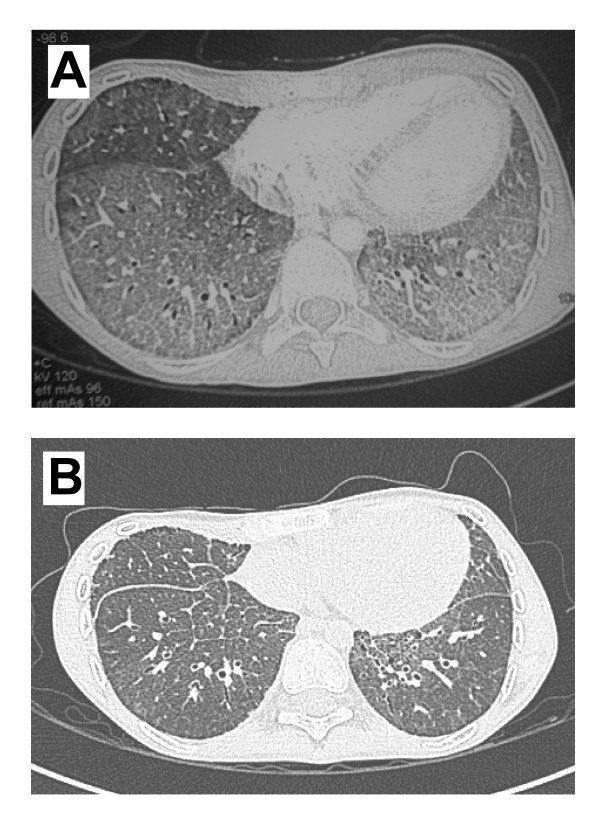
Chest HRCT. Panel A. Lung CT scan obtained before WLL (December 2008). Bilateral, diffuse ground glass of lower pulmonary lobes, with thickening of intra- and inter-lobular septa (crazy paving pattern). Panel B. Lung CT scan obtained after inhaled rGM-CSF trial (April 2010), showing a marked bilateral improvement in lower lobe involvement; nevertheless, lung density appears still increased.
